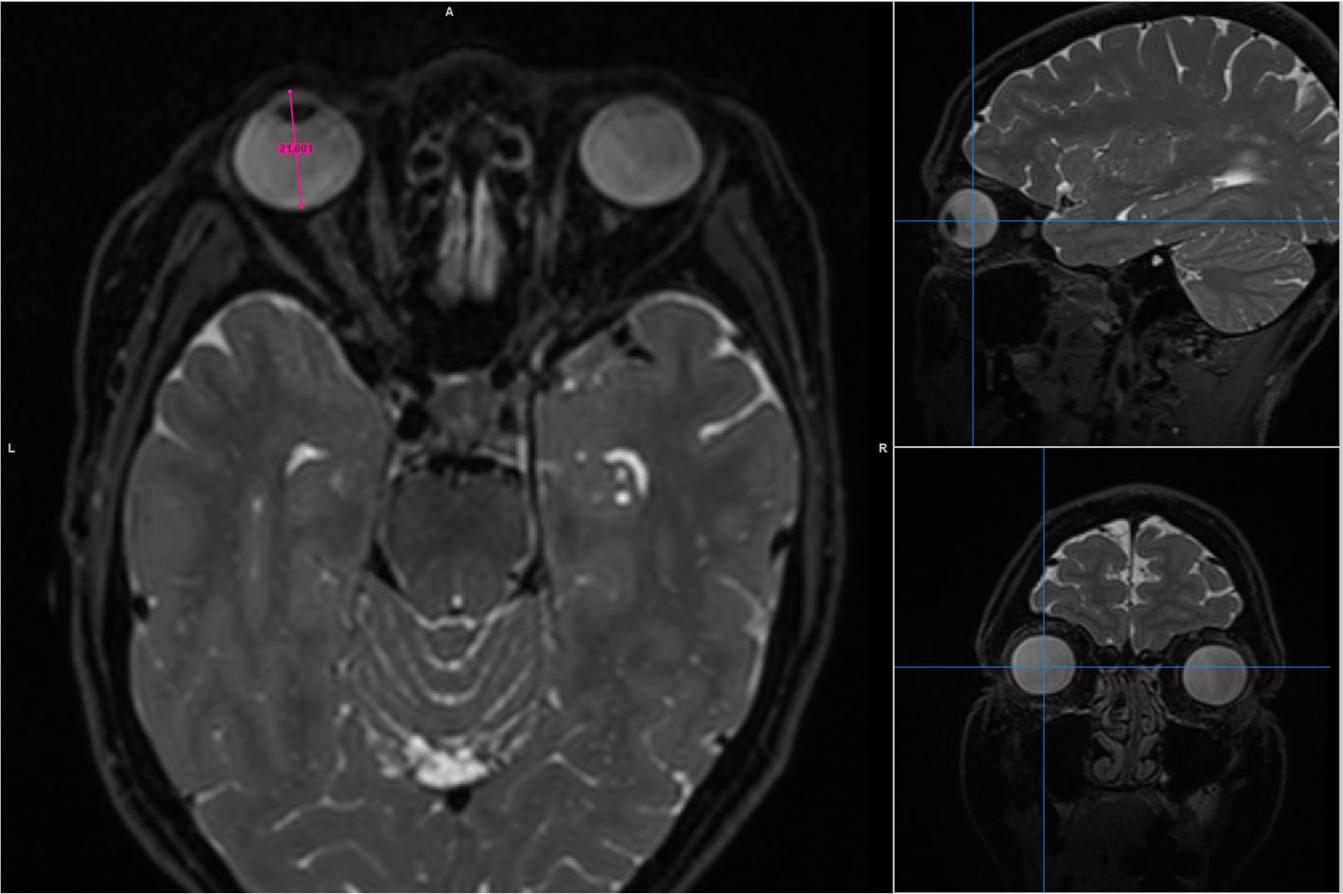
Procedure for finding the centre of the eye in three orthogonal planes on T2-weighted brain MRI. Example shown is the Mango image analysis tool – the main image component displays the brain in an axial orientation with two supplementary projections (sagittal top and coronal bottom) used to guide the user to the centre of the globe. Zoom tools were then used on the axial image to better visualize the anterior and posterior boundaries of the globe. Maximum eye axial length is then measured on the axial image (pink line). Supplementary Figs. 2, 3, 4, 5, 6, 7, 8 show examples using the Caresteam image analysis tool, magnified on the axial image to better show the anterior and posterior measurement boundaries
